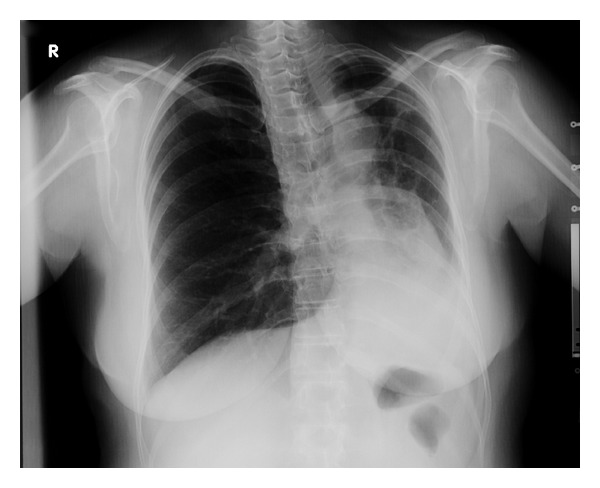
Chest radiograph showing loss of volume of her left lung with displacement of the mediastinum to the left.
